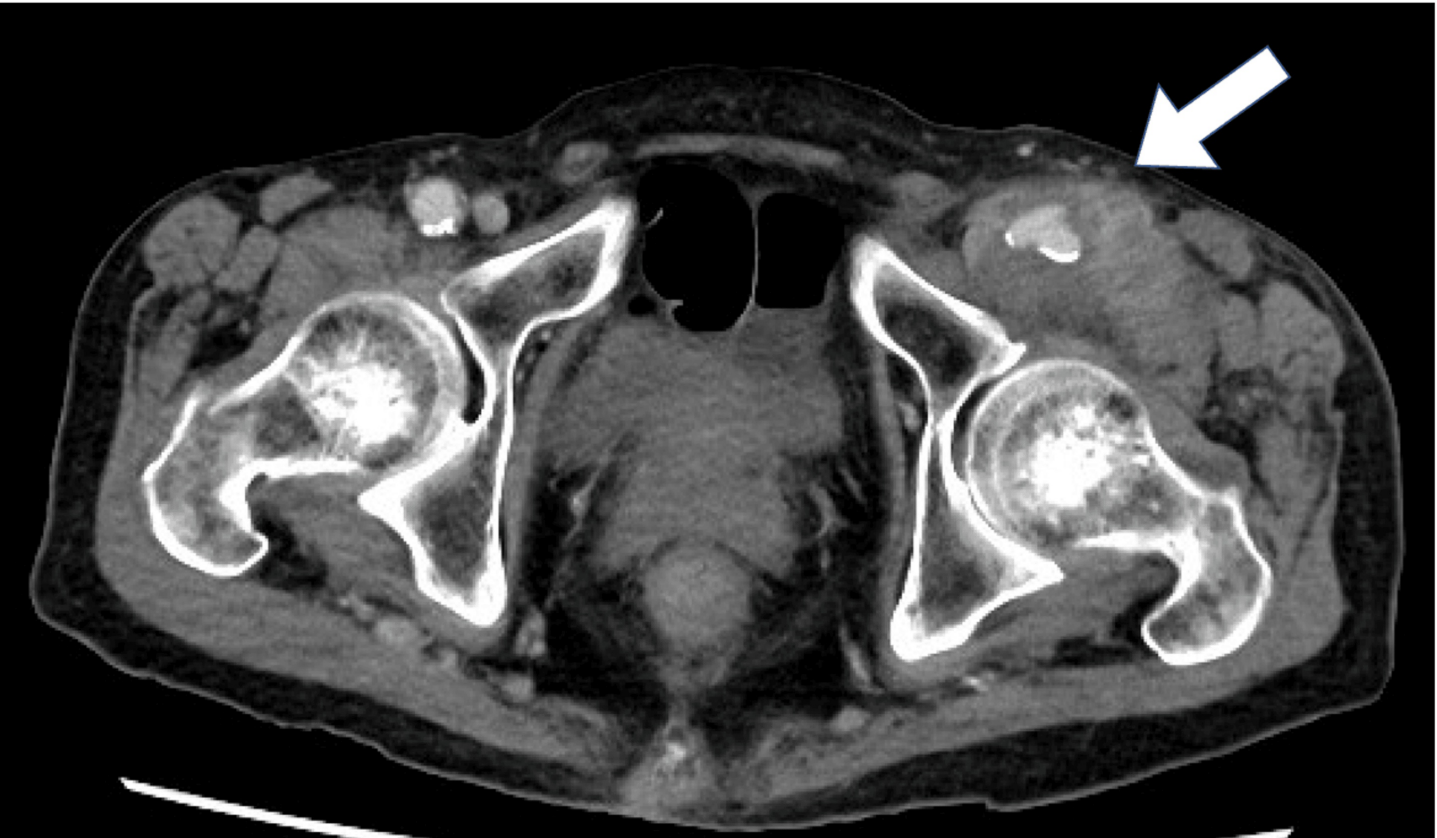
The previous hospital's enhanced CT shows a pseudoaneurysm with diameter of 41 x 50 mm The arrow indicates the failed vessel wall and the leaking blood, which is compressing the surrounding tissue. CT: computed tomography
